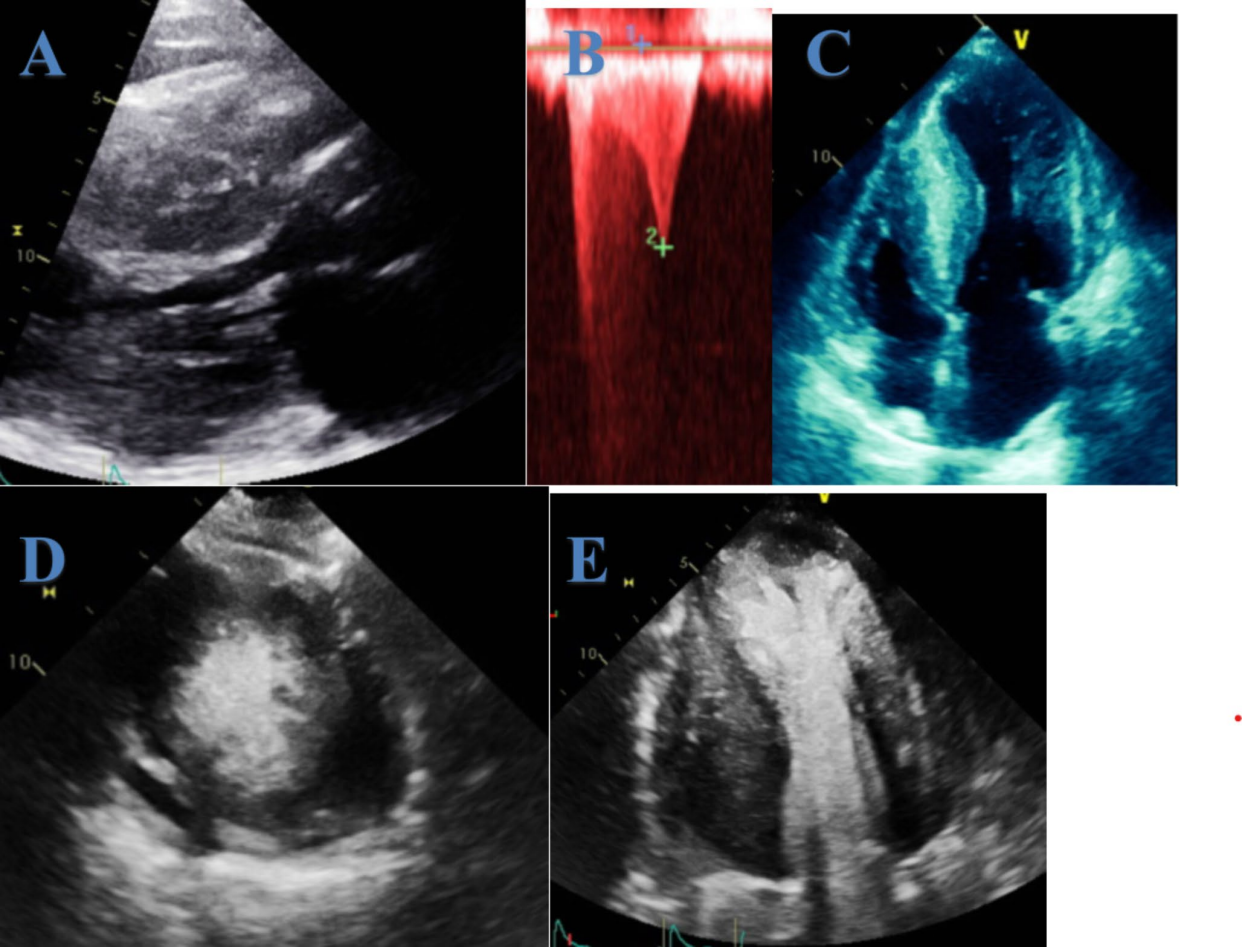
Echocardiography of a patient with hypertrophic obstructive cardiomyopathy. A: Parasternal long-axis view showing a thickened left ventricular wall. B: Doppler of the left ventricular outflow tract (LVOT) reveals a characteristic dagger-shaped signal with a LVOT gradient of 64 mmHg. C, D, and E: Thickened ventricular walls are shown, with and without contrast enhancement
